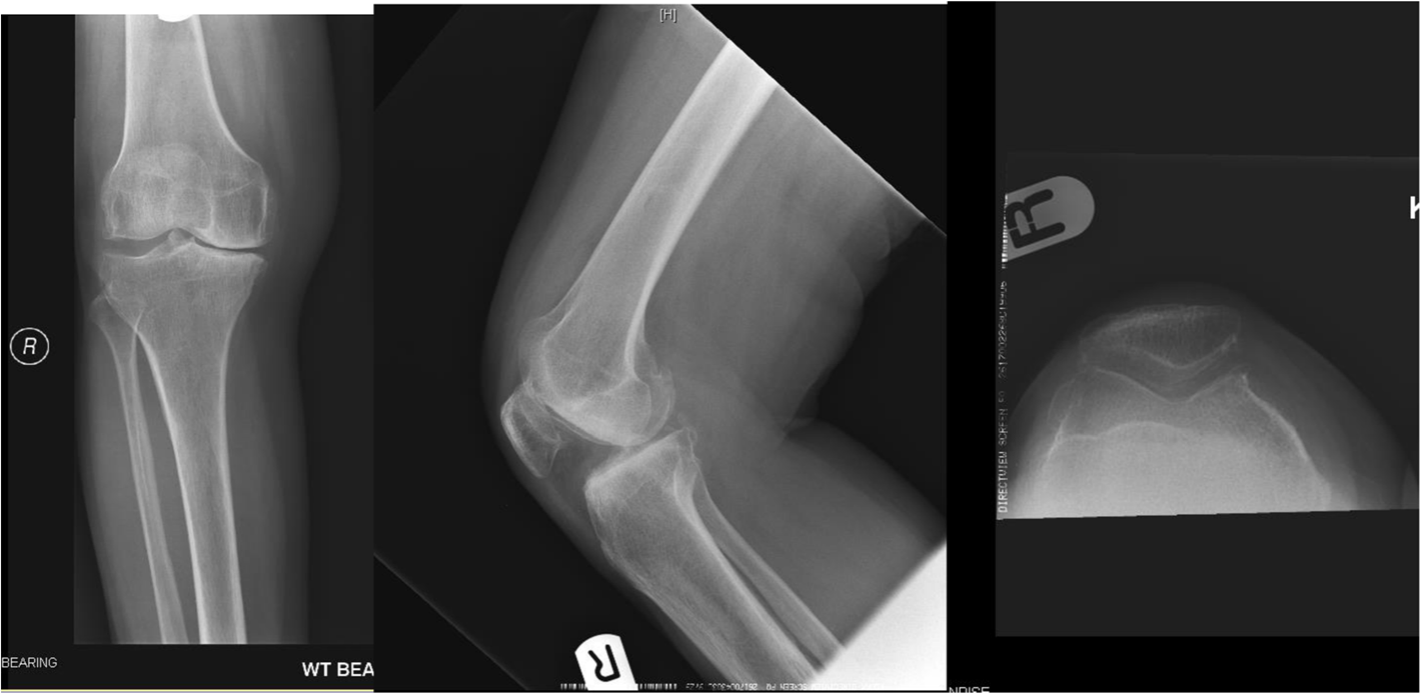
Pre-operative radiographs of her knees
